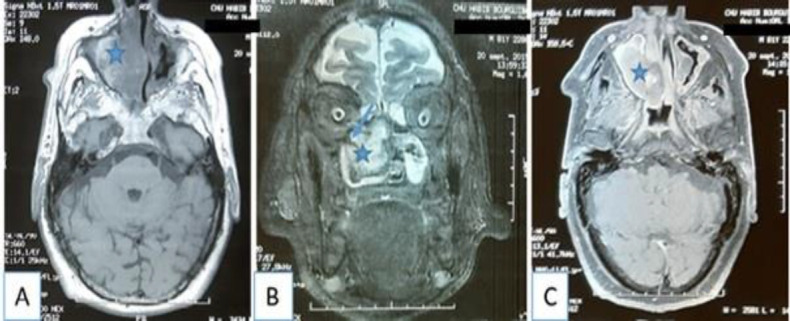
MRI of the parasansal sinuses: (A) axial section of T1-weighted: a well-defined expansible mass of the right maxillary sinus and the nasal cavity with low signal intensity. (B) coronal section of T2-weighted: the mass demonstrates heterogeneous high signal intensity with extension into the right orbit (arrow). (C) axial section of contrast-enhanced MRI: heterogeneous enhancement of the mass
